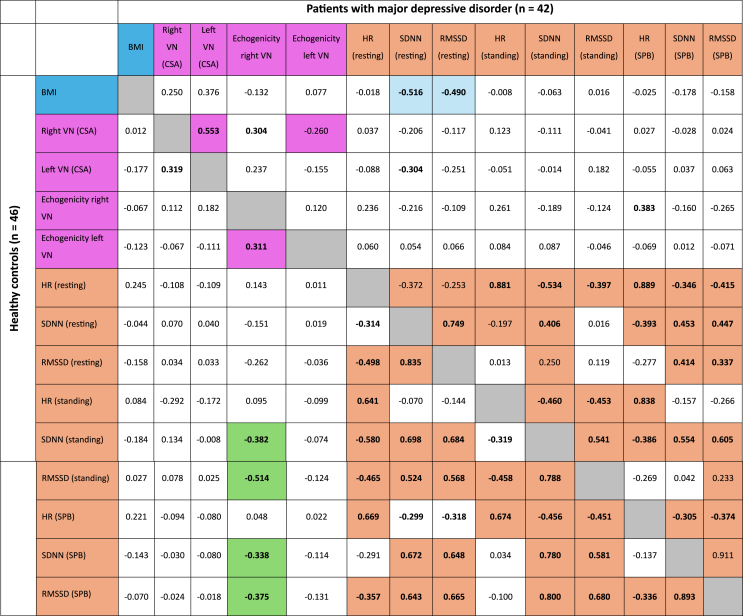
Correlation coefficients (rho) of vagus nerve (VN) high-resolution ultrasound parameters (cross-sectional area (CSA) and echogenicity) and heart rate variability parameters in 42 patients with major depressive disorder (upper part of the table, above the grey diagonal) and in healthy controls (n = 46, lower part of the table, below the grey diagonal). Participants with cardiac arrhythmia and/or intake of beta-blockers were excluded from heart rate variability analyzes. Significant results (2-tailed) of the bivariate correlation (Spearman) are given in bold, while significant results (2-tailed) of the partial correlation with controlling for the body mass index (BMI) are given with a colored background. SDNN standard deviation of RR-intervals, RMSSD root mean square of successive differences, SPB slow paced breathing, Sig significance.
